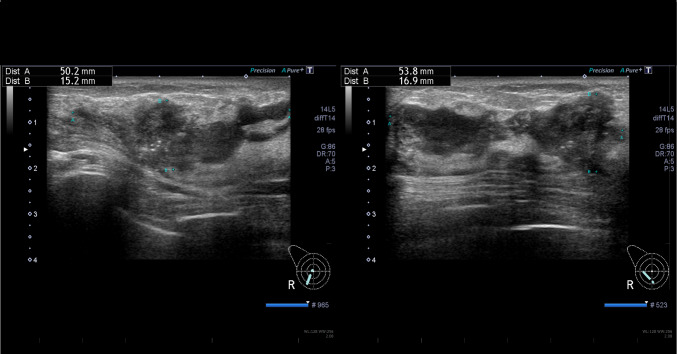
Ultrasonography. Tumor with ill-defined, irregular shape and low echogenicity was detected
